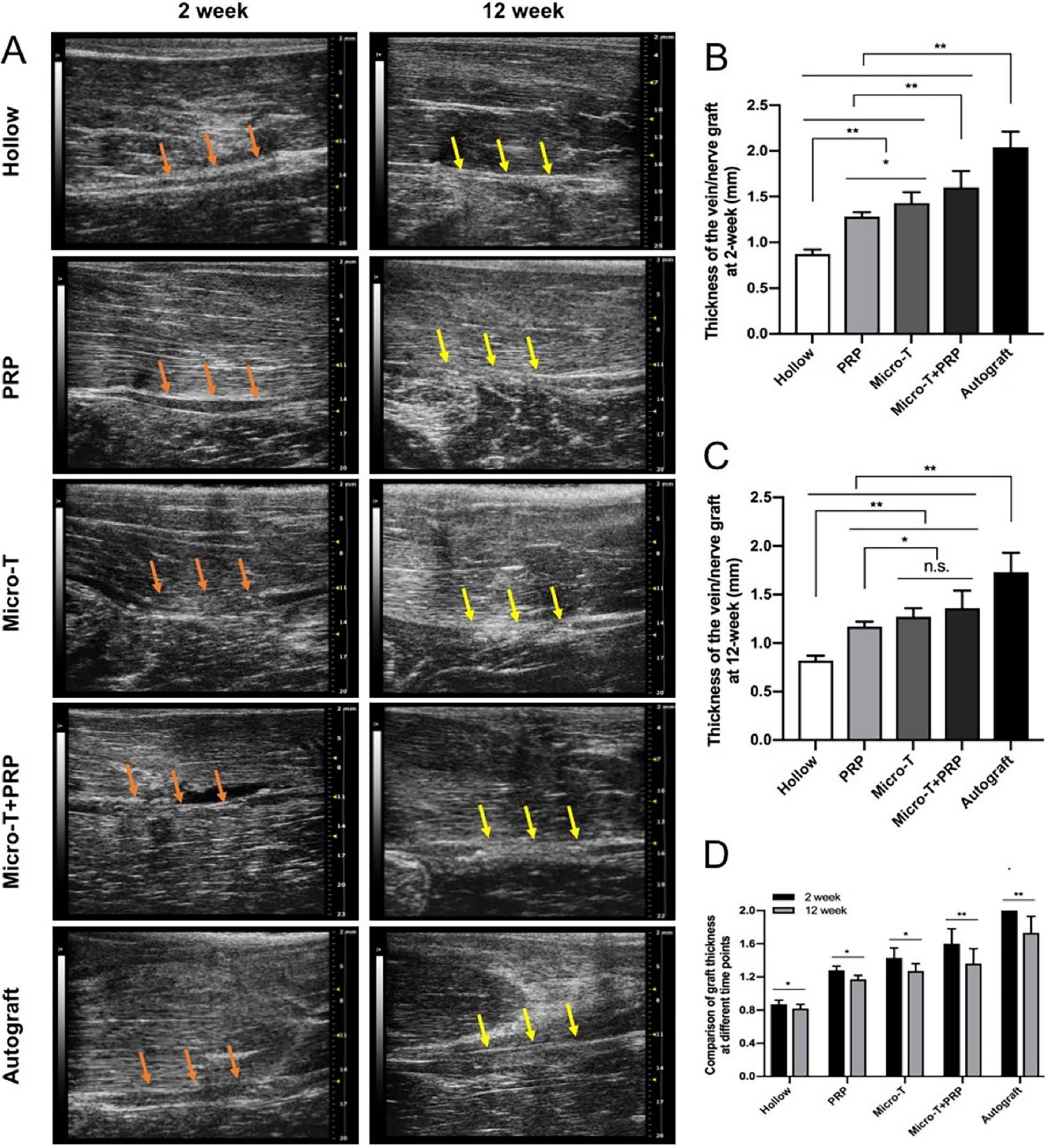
High-frequency nerve ultrasound examination was performed at 2 weeks and 12 weeks after operation. A High-frequency ultrasound images of the nerve graft on the surgical side. Statistical analyses were performed by calculating the thickness of the nerve graft at B 2 weeks and C 12 weeks. D Comparison of nerve graft thickness at different time points. ^^*P* < 0.01, and n.s. not significant. Micro-T microtissues, PRP platelet-rich plasma
